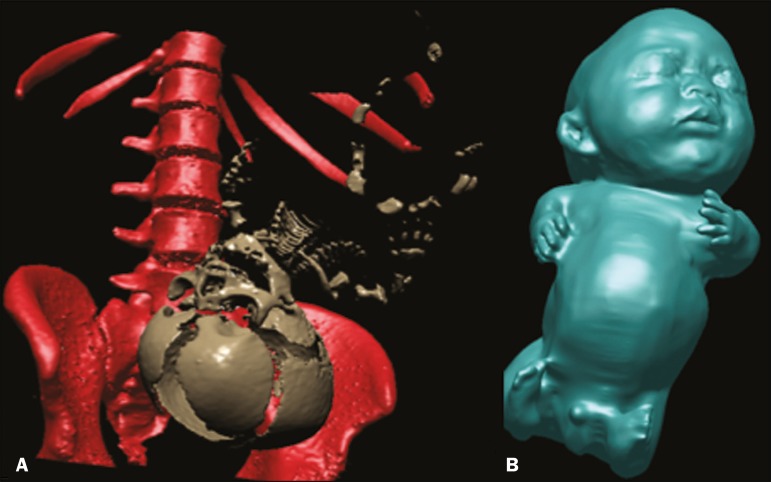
Achondrogenesis at 34 weeks of gestation. A: 3D view from a CT scan. B: 3D view from an MRI scan.
